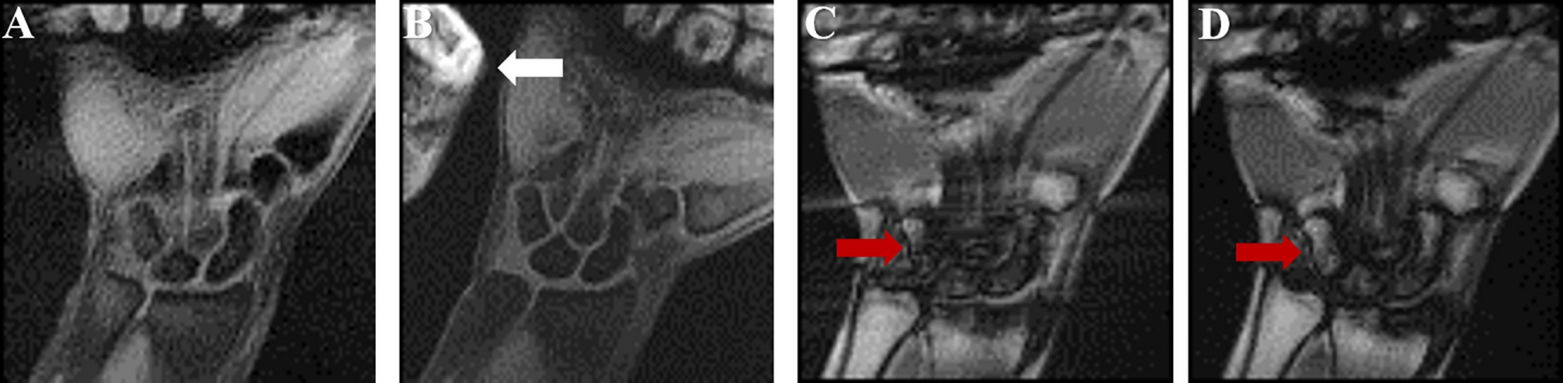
Comparison of real-time MRI pulse sequences, namely (a) radial-fast GRE, (b) Cartesian-fast GRE, (c) radial-bSSFP, and (d) Cartesian-bSSFP from study participants during radial-ulnar deviation. White and red arrows point to the wraparound effect and banding artifacts, respectively.
